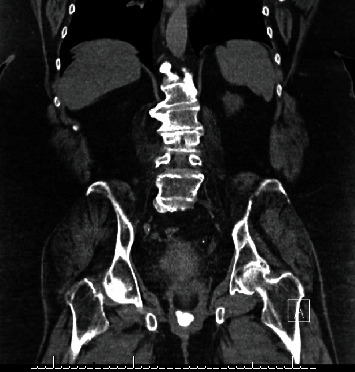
Coronal CT scan image of pelvis showing calcified vaginal mass.
